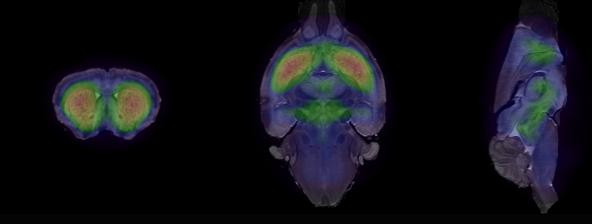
Coregistered template. In building the template, the anatomical areas are confirmed by coregistering the template to an available MRI brain template
